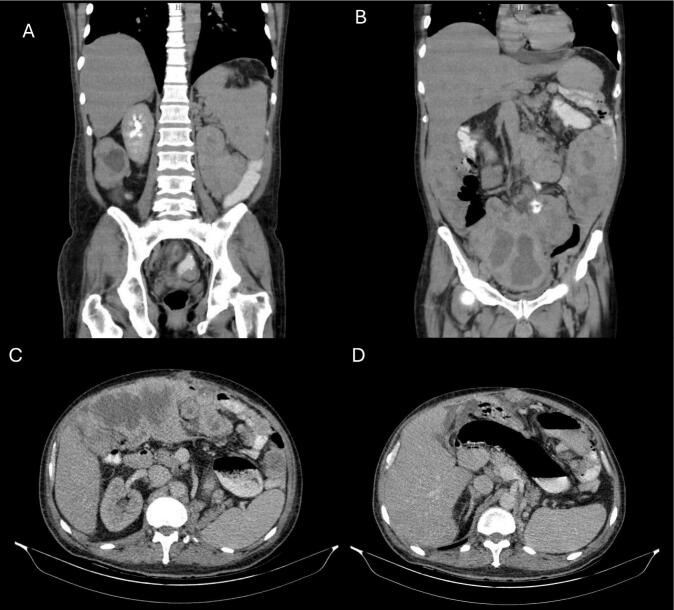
Abdominopelvic CT scan of liposarcoma case 3. A,B: coronal view displaying the extent of the liposarcoma in the vertical plane. C: axial view showing the transverse cross-section of the liposarcoma occupying abdomino-pelvic cavity was seen with the size of 350*165 from the umbilical area to the right. D: axial view showing the transverse cross-section of the liposarcoma and in incasement of left kidney. Another mass in the LUQ was seen with a previous size of 68*53.
